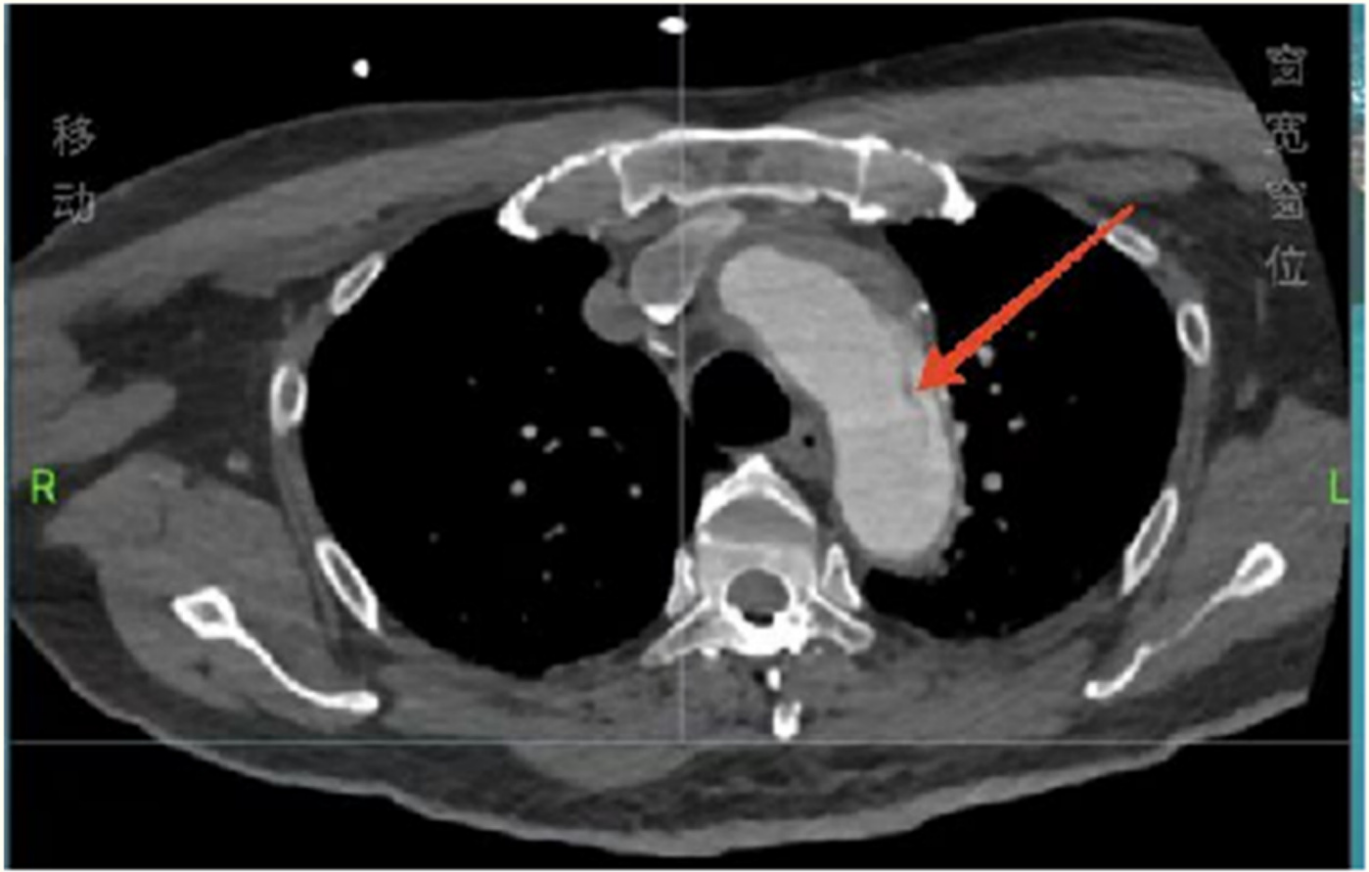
Total aortic computed tomography angiography showing an aortic arch dissection.
